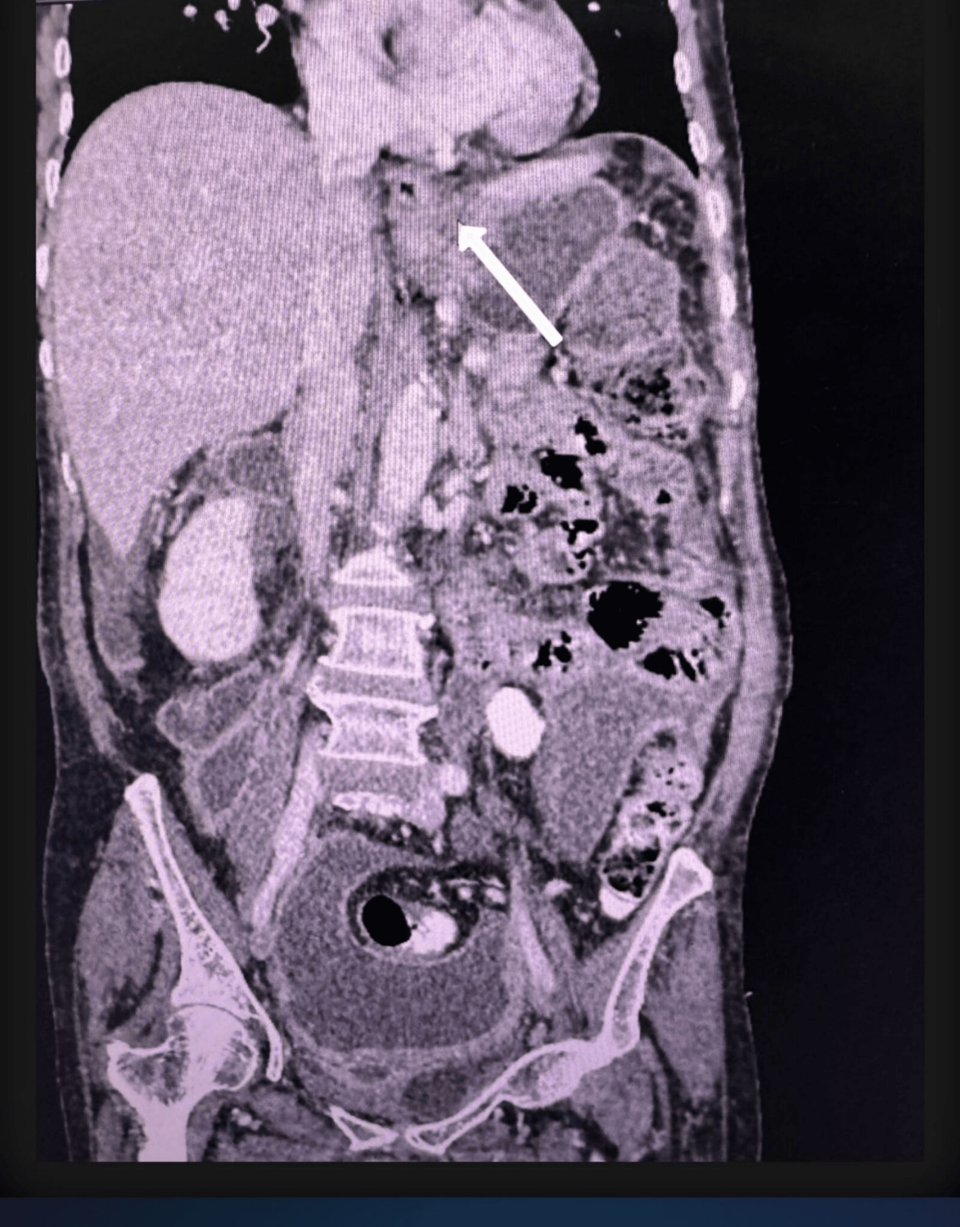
CECT axial section revealing the GE junction mass extending to cardia (arrow) CECT: contrast-enhanced computed tomography; GE: gastroesophageal
